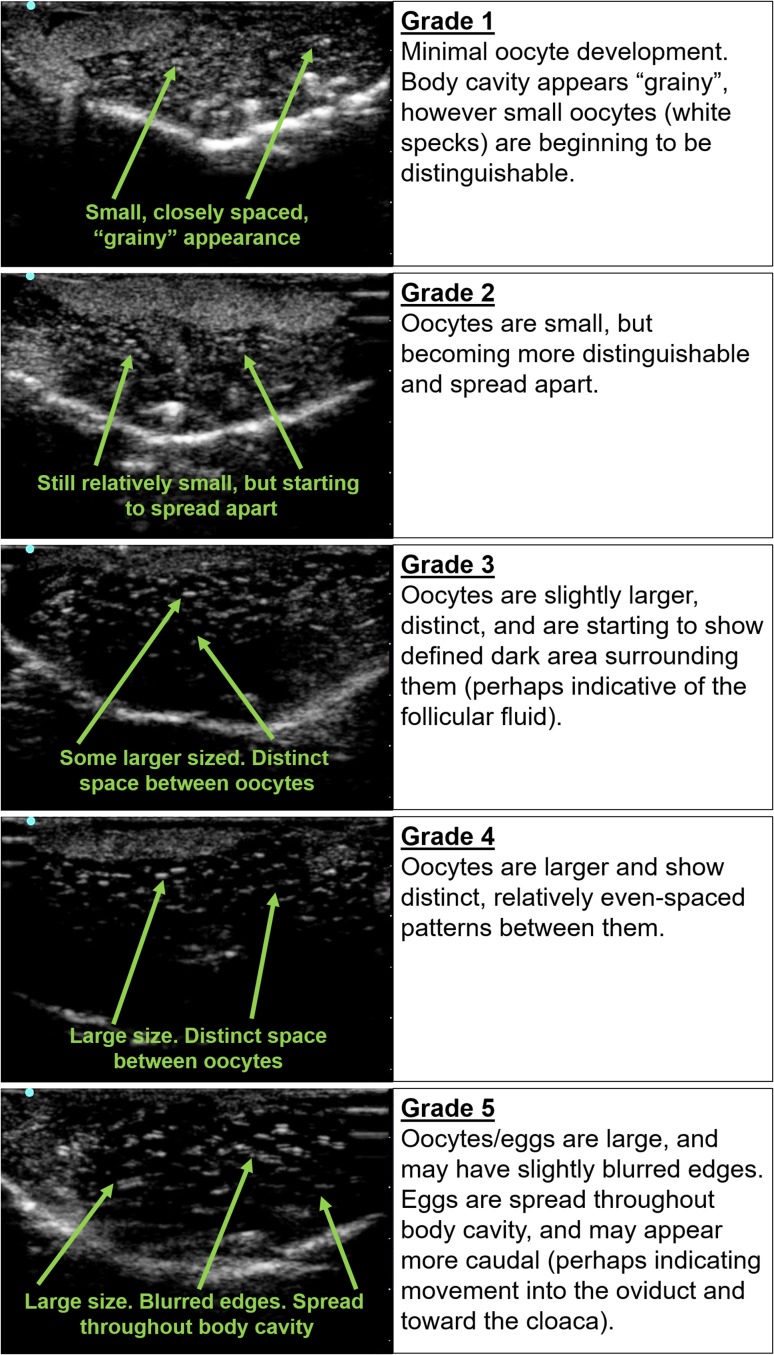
Transabdominal ultrasound grading scale (Grades 1–5) for dusky gopher frogs.
